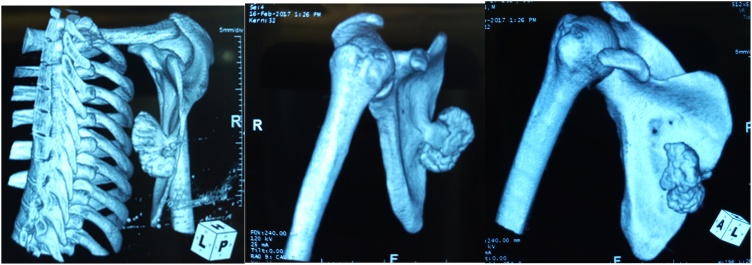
CT scan 3D reconstruction of the right scapula showing exostosis.
